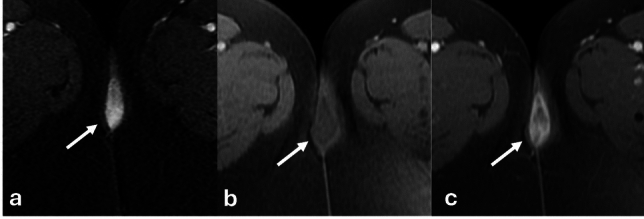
21-Year-old female with labial mass. Axial fat-saturated T2-weighted images (a) and pre- and post-contrast T1-weighted images (b-c) demonstrate a uniformly T2 hyperintense, heterogeneously enhancing mass (arrow) at the midline vulva. The patient underwent surgical resection, with pathology showing angiomyofibroblastoma
